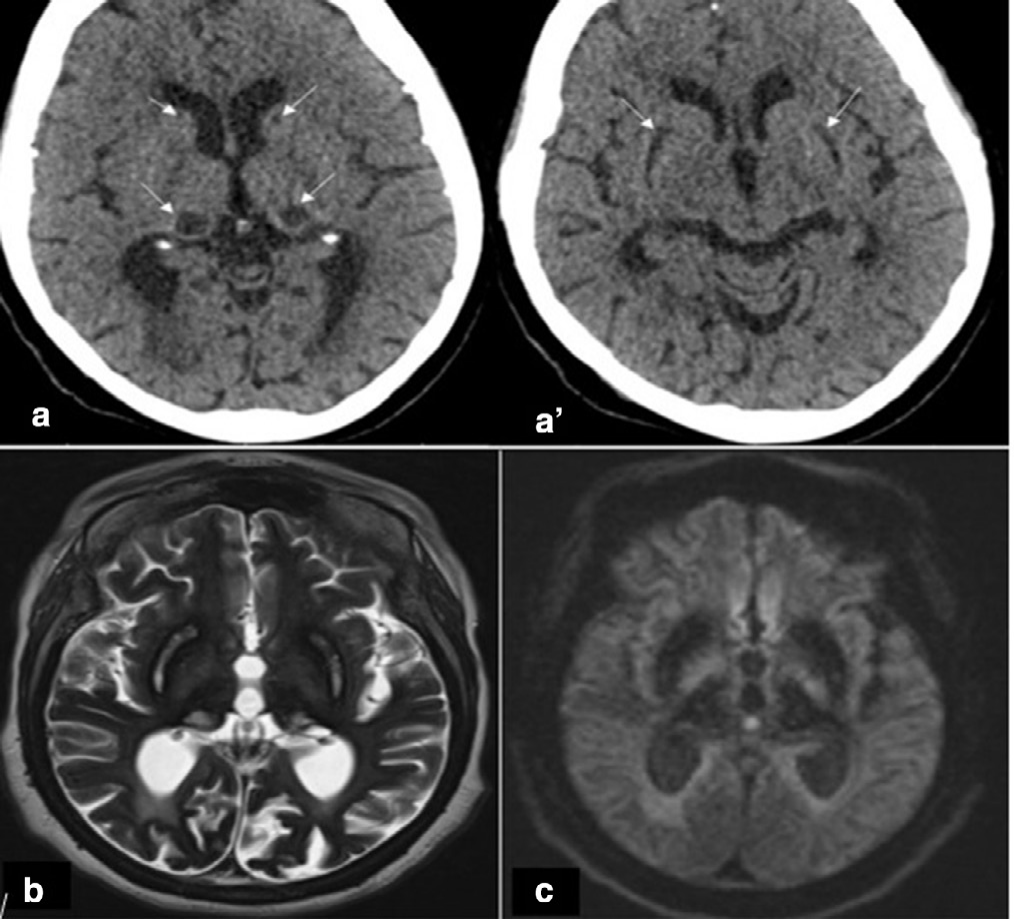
(A and A’) Axial CT scan showing hypodensity in the basal ganglia bilaterally (putamen, thalami and caudate). (B, C) Brain MRI (1,5 T): (B) Axial T2 showing hyperintense T2 signal in areas that were hypodense on CT surrounded by hypointense with also diffuse and bilateral hypointense cortices. (C) Axial diffusion showing hypo signal diffusion
